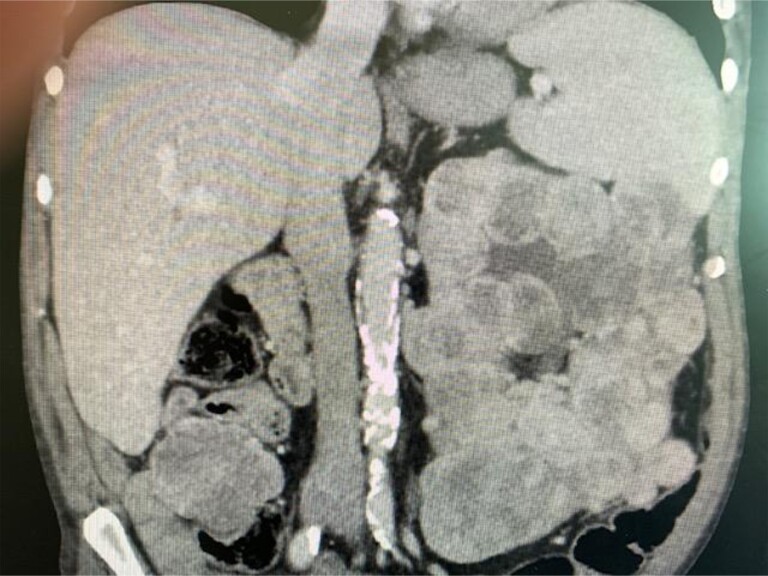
CT imaging of the abdomen and pelvis obtained after the administration of intravenous contrast material revealed a 5 × 5.4 × 18.2-cm lobulated mass infiltrating the left kidney, which was thought to be the putative source of IGF-2 excess.
